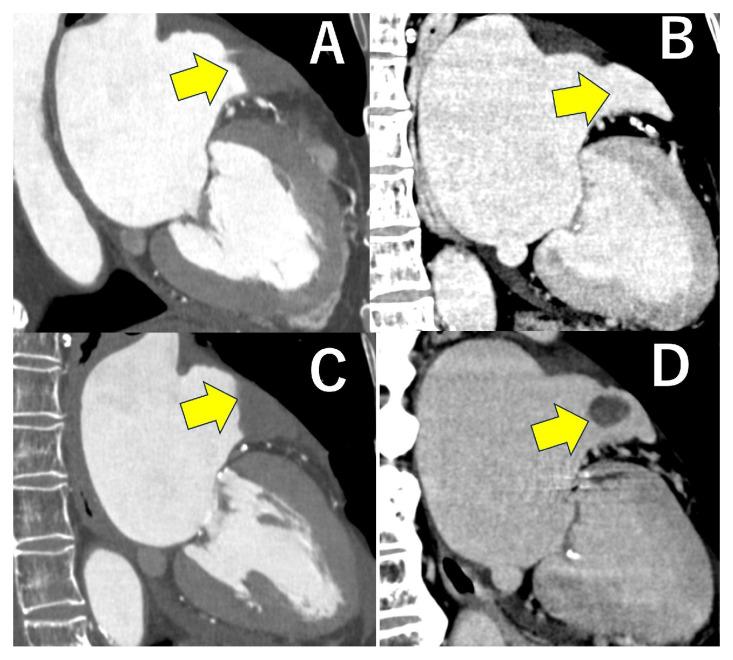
In cardiac computed tomography of patients with atrial fibrillation, delayed contrast agent filling into the left atrial appendage may be observed during the early phase of coronary artery evaluation. In such instances, employing additional late phase contrast images to assess both myocardial injury and left atrial appendage thrombus is highly significant. Upper panel: In the early phase, the contrast agent did not reach the tip of the left atrial appendage, making differentiation from a thrombus difficult (A). However, in the late phase, contrast was observed up to the tip, confirming the absence of a thrombus (B). Lower panel: Similarly, differentiation was difficult in the early phase (C). However, late phase imaging revealed the presence of a thrombus (D) (yellow arrows).
